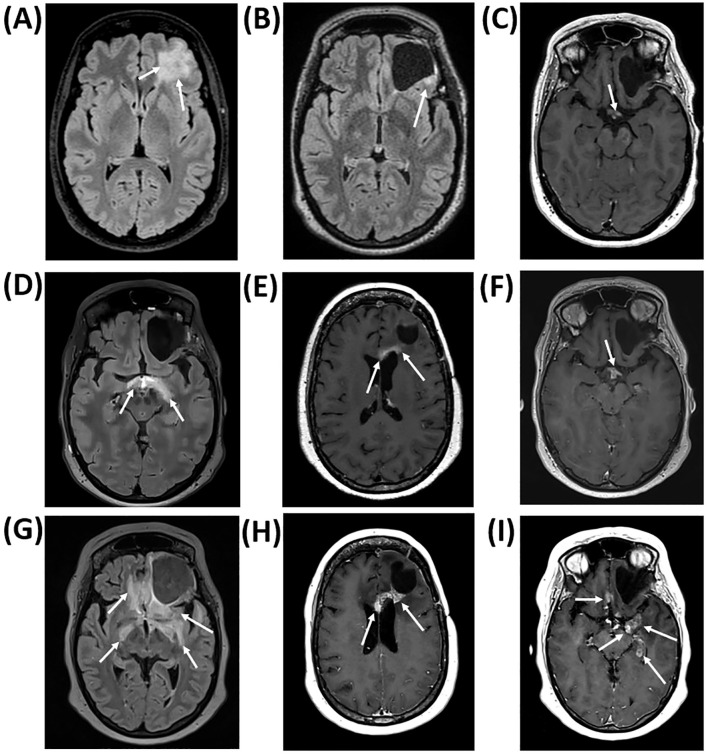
Axial magnetic resonance imaging (MRI) scans. (A) displays a left-sided, frontal, diffuse T2-weighted fluid-attenuated inversion recovery (FLAIR) hyperintense and gadolinium non-enhancing lesion in the preoperative MRI scan. (B) Postoperative T2-weighted/FLAIR image showing a small residual tumor (10 millimeters) dorsolateral to the resection cavity. (C) displays subtle chiasmatic contrast enhancement in a T1-weighted MRI-series at symptom debut 9.5 months following completion of radiotherapy, (D) shows FLAIR hyperintensity approximately one month after symptom debut, and (E, F) show contrast enhanced T1-weighted image sequences six months from symptom debut, with radiation-induced contrast enhancement in optic pathways and genu corporis callosi. (G–I) display T2-weighted/FLAIR hyperintensity changes and increasing contrast enhancement in an MRI scan 12 months from symptom debut and two weeks before the patient passed away, corresponding to 21 months after radiotherapy completion. Arrows are used to draw attention to pathology in all images.
